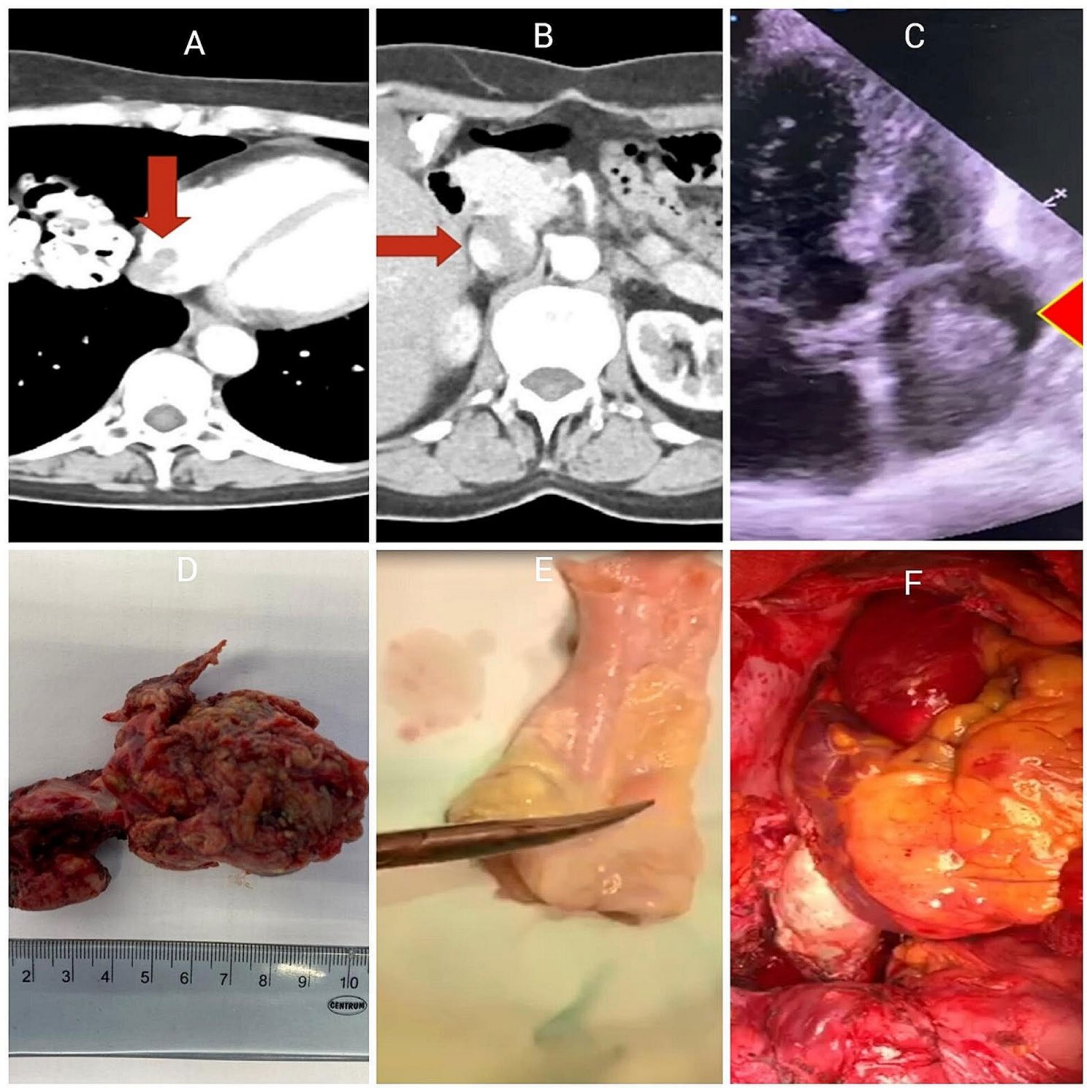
CT scan before operation, tumor trombosis into right atrium and inferior vena cava (A,B), TEE view before operation demonstrate tumor mass into right atrium (C), resected part of IVC and right atrium (D), cryopreserved pulmonary homograft 28 mm (E), final view (F)
